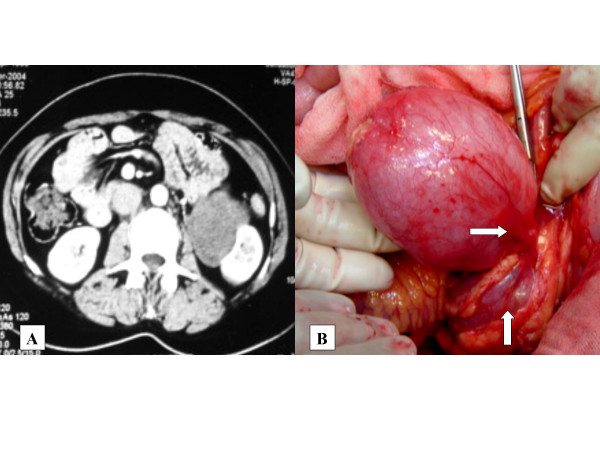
A. CT scan showing homogenous mass located at medial aspect of the left kidney and compressing it with minimal contrast enhancement. B. Intraoperative picture demonstrating the mass which is free from renal parenchyma (vertical arrow) and attached to renal pelvis with a narrow pedicle (horizontal arrow)
